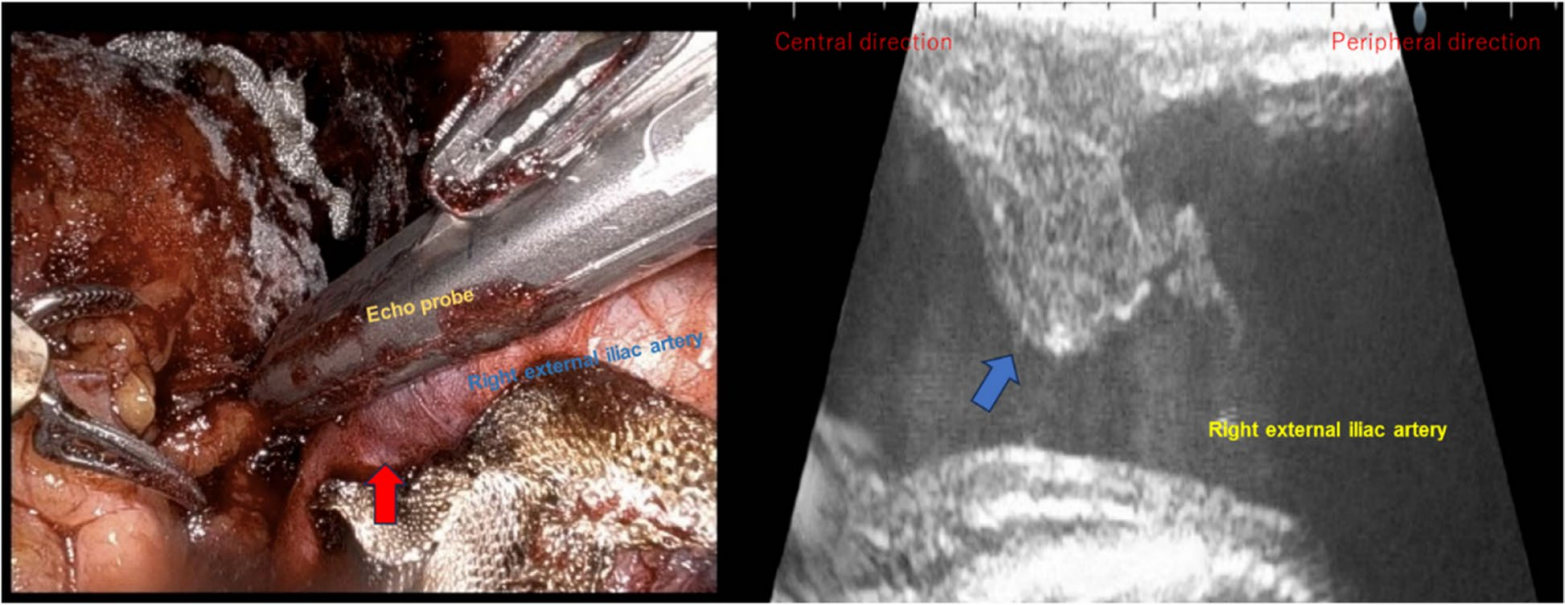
The right external iliac artery has a darker tone (red arrow). Ultrasound imaging shows an intramural hematoma with partial disruption on the ventral side of the external iliac artery lumen (blue arrow)
